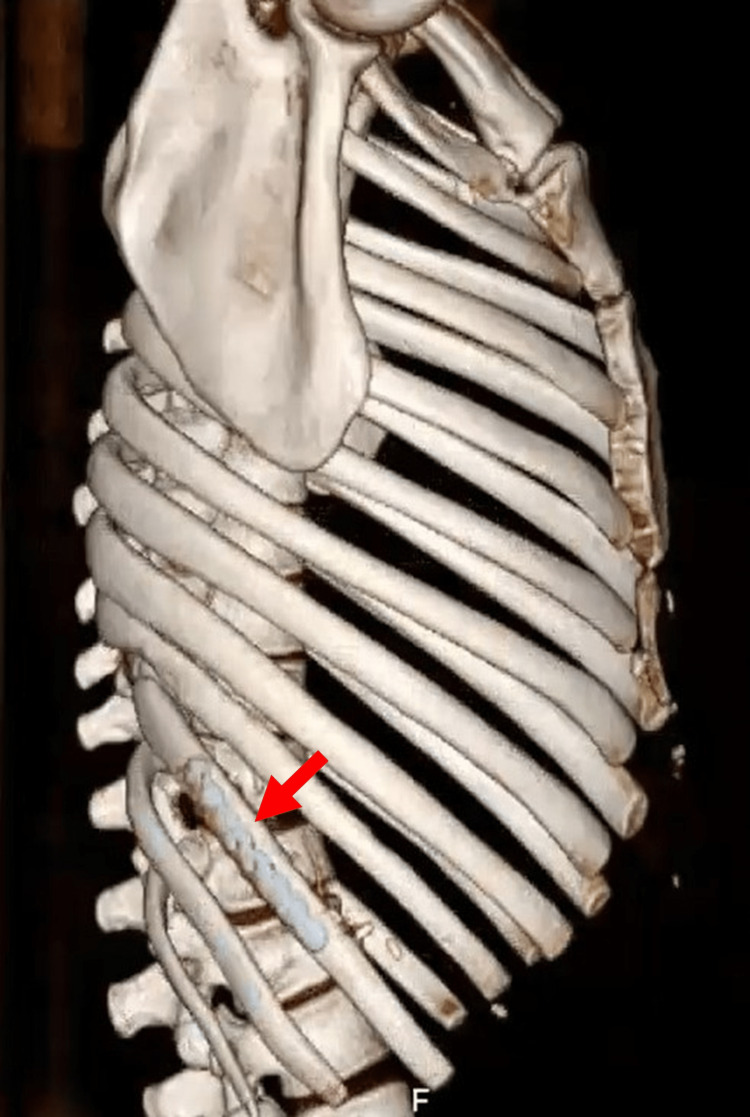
Three-dimensional CT reconstruction showing the osteosynthesis of the 10th fractured rib using the MatrixRIB® system (red arrow).
